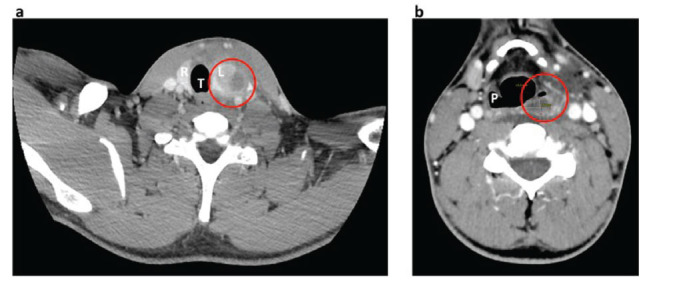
Contrast enhanced neck computed tomography. (a) Transversal section including thyroid. An inhomogeneous area with a large hypodense portion of colliquated appearance can be observed in red circle, involving the upper pole of the left thyroid lobe, and accompanied by significant oedema of the surrounding adipose tissue. (b) Transversal section at the level of the pharynx. Obliteration of the left pyriform sinus can be observed in the red circle. T=trachea, I: thyroid isthmus, L=left thyroid lobe; R= right thyroid lobe, P=pyriform sinus.
